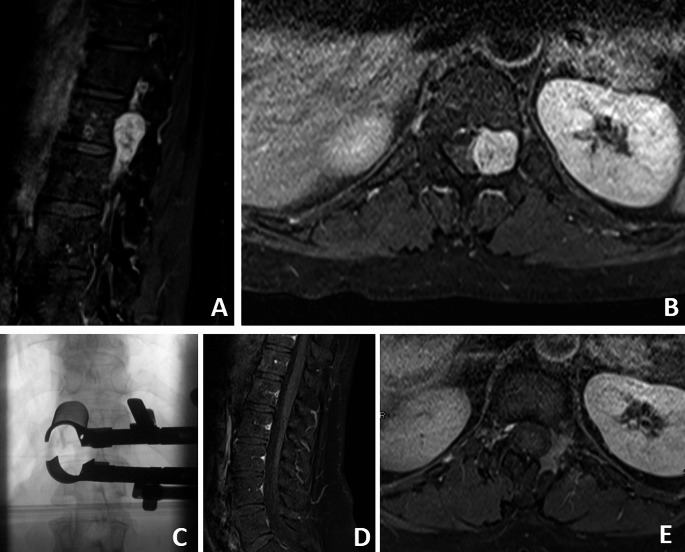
Exemplary case of an IVa classified dumbbell schwannoma (case 34). The expansion of the tumor intraforaminal is shown on the axial MRI scan (B). The extradural tumor mass is shown on the sagittal scan (A). Intraoperative X-rays of patient 34 show the retractor placement from anterior-posterior (C). Postoperative sagittal (D) and axial (E) MRI scans of patient 34 show a GTR of the IVa classified dumbbell schwannoma while sparing > 50% of the facet joint
